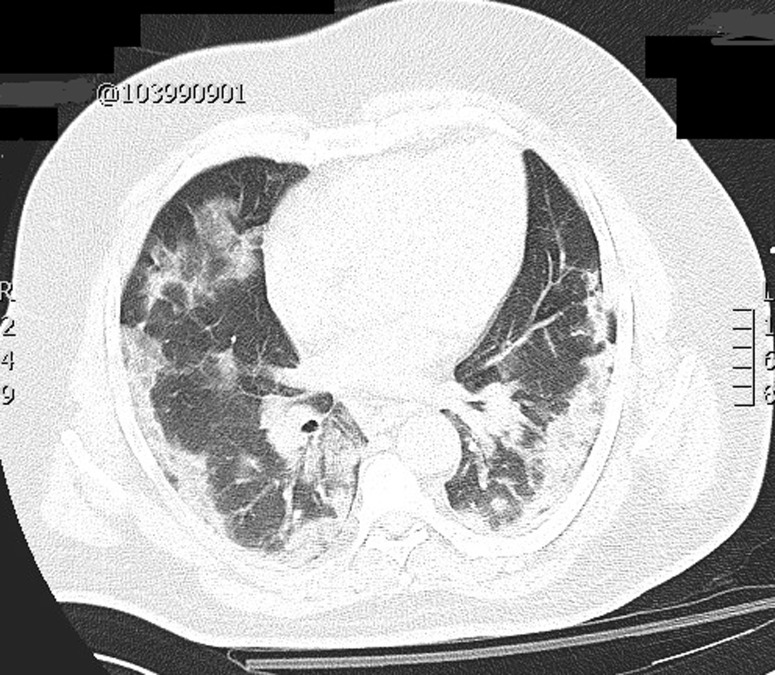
chest CT of a patient COVID positive with the typical interstitial pneumonia
